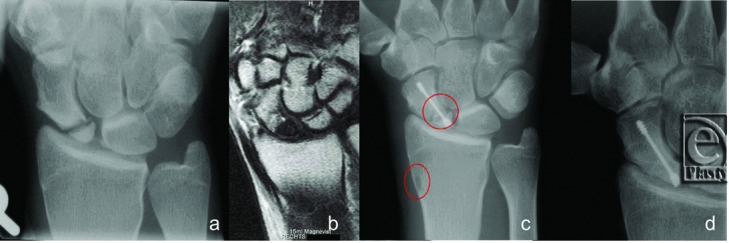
X-rays of a proximal located scaphoid nonunion. A 25-year-old male patient suffering from scaphoid nonunion for 1.5 years. (*a*) Preoperatively. (*b*) Preoperative MRI with avascular proximal fragment. (c) Intraoperative aspect with the mini-Herbert screw after dorsal incision, red marked is the harvesting location of the bone graft and its new location in the nonunion site. (d) X-ray investigation after 12 weeks.
